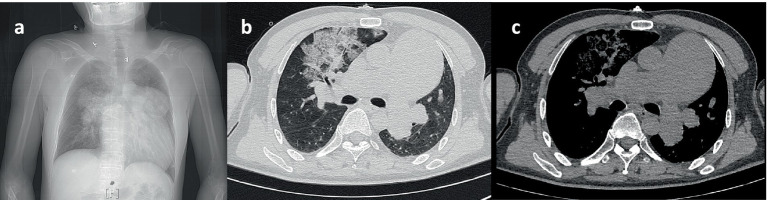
(a–c) The chest computer tomography (CT) scan displayed a double lung infection.
